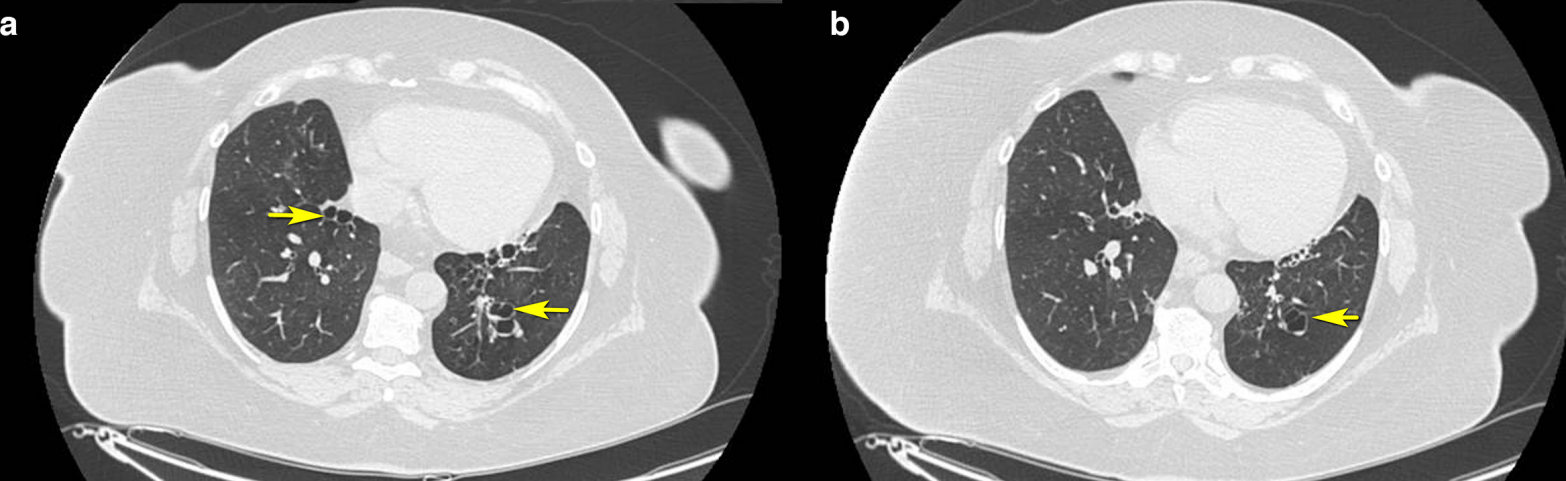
A and B. Axial non-contrast CT images of the chest in expiration showing the collapse of the bronchiectasis indicating the absence of cartilaginous plates in subsegmental bronchi.
